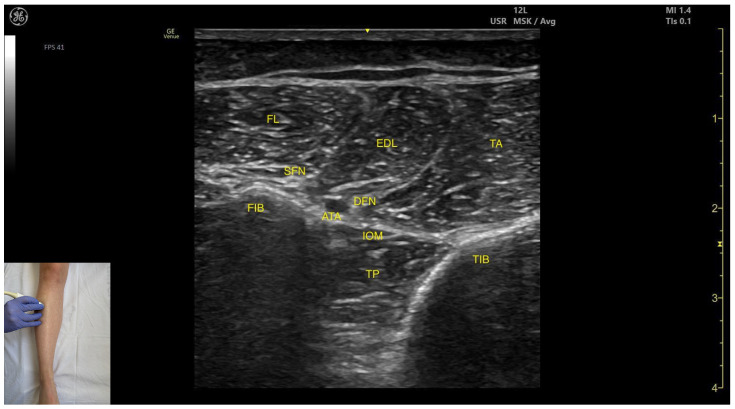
Ultrasound anatomy of the extensor digitorum longus: FIB—fibula; FL—fibularis longus; SFN—superficial fibular nerve; ATA—anterior tibial artery; DFN—deep fibular nerve; EDL—extensor digitorum longus; IOM—interosseous membrane; TP—tibialis posterior; TA—tibialis anterior; TIB—tibia.
